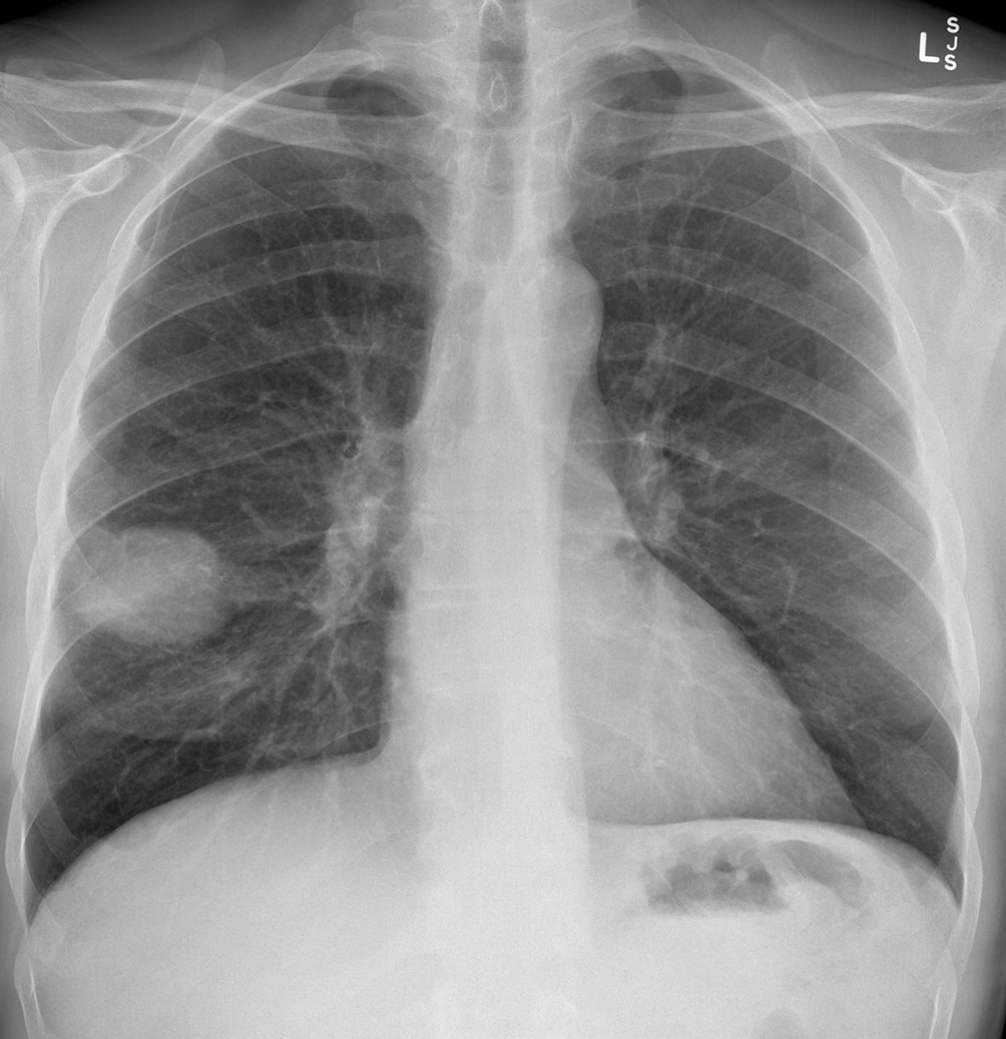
A large right middle lobe mass is identified on screening chest radiograph. On revisitation of history, 22 kg of unintentional weight loss over 3 months and a 40 pack-year smoking history were revealed.
